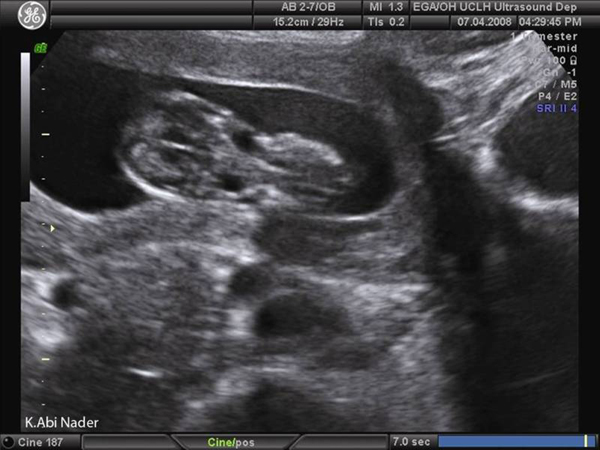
Coronal section: A coronal ultrasound section of the fetal head and neck region at 13 weeks of gestation shows bilateral cysts in the middle-half of the neck.
